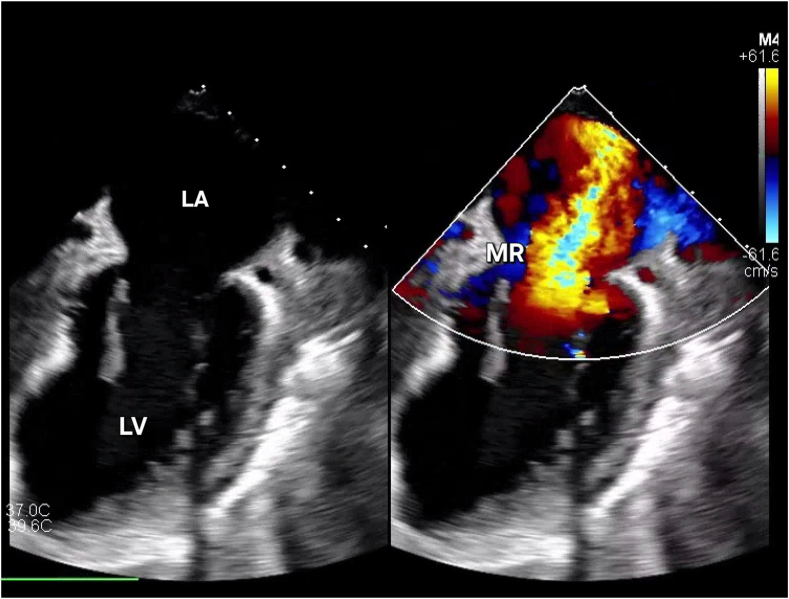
Transthoracic echocardiogram (on presentation) showing severe MR. LA, left Atrium; LV, left ventricle; MR, mitral regurgitation.
